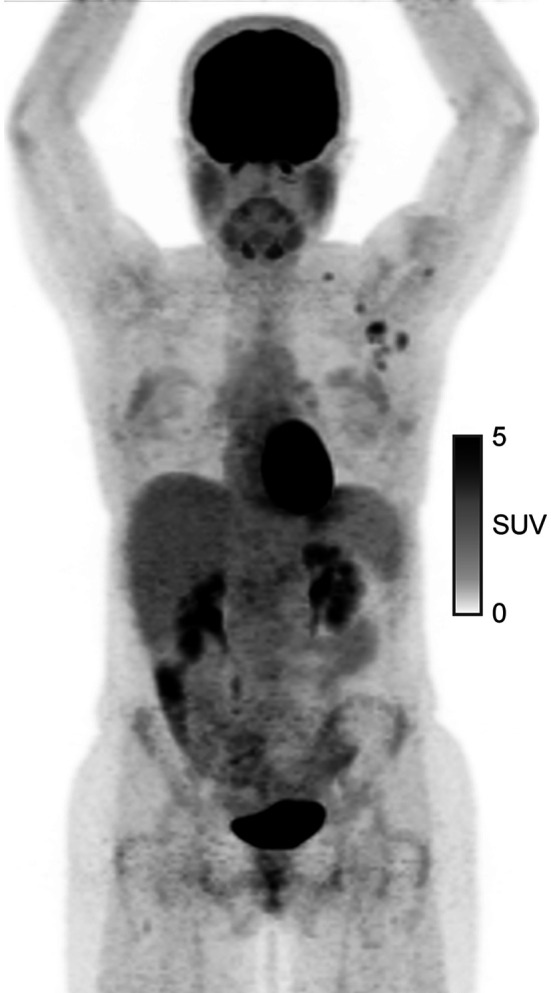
^18^F-FDG PET/CT showing left axillary and left supraclavicular avidity. Maximum-intensity-projection image with SUV scale at right.
